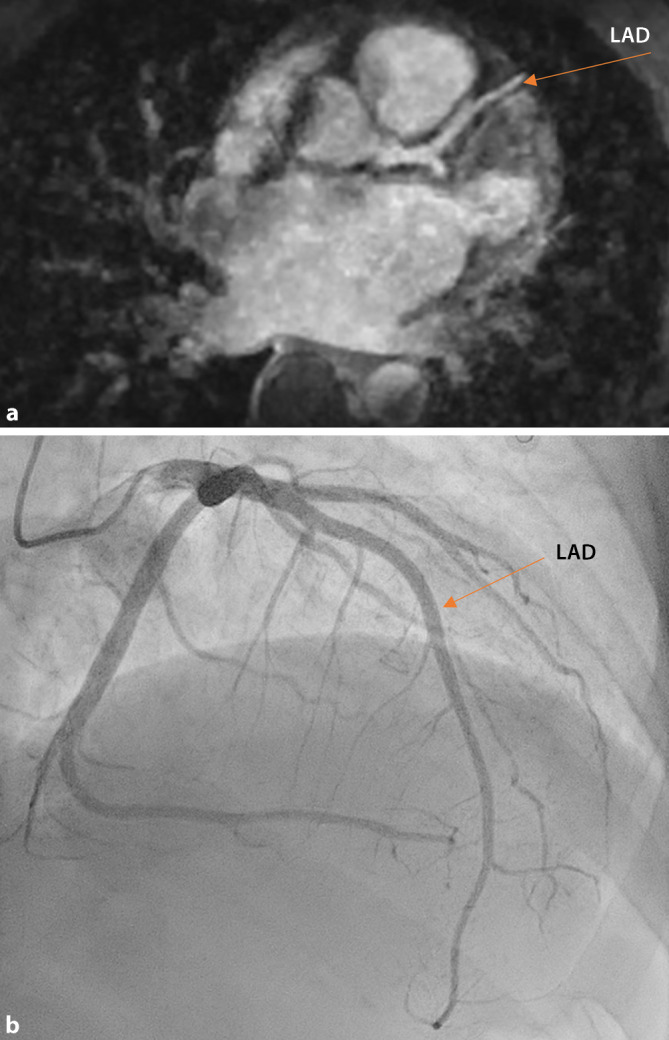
Coronary imaging using invasive coronary angiography and the whole-heart sequence in coronary magnetic resonance angiography (CMRA) for visualization of the coronary arterial tree. Self-navigated 3D CMRA. Whole-heart sequence (CMRA, a) with corresponding image from the invasive coronary angiography (coronary computed tomographic angiography, b) acquired from a patient revealing the proximal left anterior descending artery (LAD; see *arrow*)
